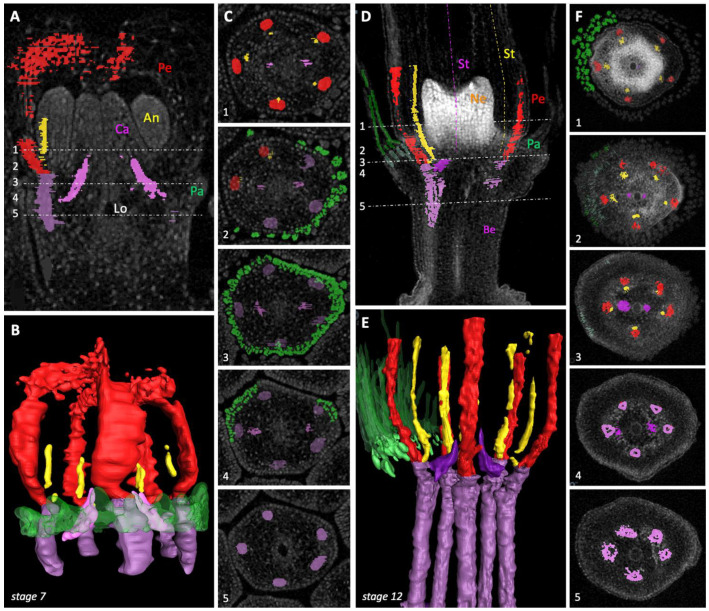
Micro-computed tomography scanning analysis of the floral vasculature and pappus development in dandelion. Young floret (*stage 7*; Table 1; (A–C)) and floret at anthesis (*stage 12*; (D–F)) presented in a vertical section (A,D), 3D-model ((B,E); Supplementary Videos SV1, SV2), and five horizontal sections (C,F) of which the cutting edges are indicated by numbers and white dashed lines in the vertical sections. Pa (green) = pappus; Pe (red) = petal bundle; An/St (yellow) = anther/stamen bundle; Ca/St (pink 1) = carpel/style bundle; (pink 2) = main bundle; Lo = locule; Be = beak; Ne = nectaries. (A) Segmentation of one of the five main bundles, its split to one of the carpels and bifurcation to a petal and an anther, and the bundle to the second carpel. (B) 3D-model showing the entire vasculature of the floret, and the pappus. Main bundles of the pappus are absent. The movie (Supplementary Videos SV1) also visualizes the bifurcation of one of the petal bundles. (C) Exact position of vascular bundles, their splits, and context to the developing pappus, confirming that the pappus initiates at the height where two main bundles split to the carpels (Number 4). (D) In mature florets, capillaries (light green) to the pappus parts (dark green) are present in the upper part of the pappus ring, and the split of bundles to the carpels and petal/anthers occur in a more compressed region (Numbers 3 and 4). Additionally, nectaries (abundant white cells) and the beak are clearly visible. (E) 3D-model showing the capillaries (solid green) to the pappus parts (green-transparent). Main bundles are much enlarged as compared to the young floret and bundles or capillaries to the nectaries are absent. The nectaries are also shown in the movie (Supplementary Videos SV2). (F) The sections show that the capillaries to the pappus parts become visible at the height where the main bundles split to the petals and anthers (Number 3). A high-resolution scan showed that they actually branch off earlier, at the bifurcation of the carpels (Supplementary Figure S3). © Monique Welten.
